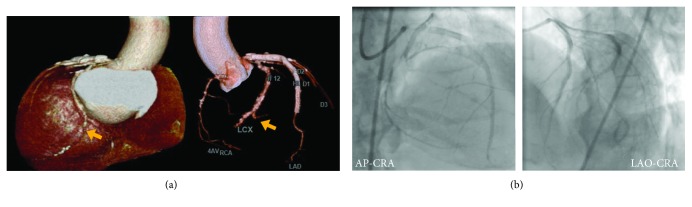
(a) Computed tomography after successful revascularization of the left anterior descending artery; the short and mildly calcified chronic total occlusion of the left circumflex artery; a stump presents after sending out the small branch (yellow arrows). (b) The middle left circumflex artery is occluded with Rentrop grade 2 collateral flow from the posterolateral branch channel and the apical channel. AP: antero-posterior; CRA: cranial; LAO: left anterior oblique.
